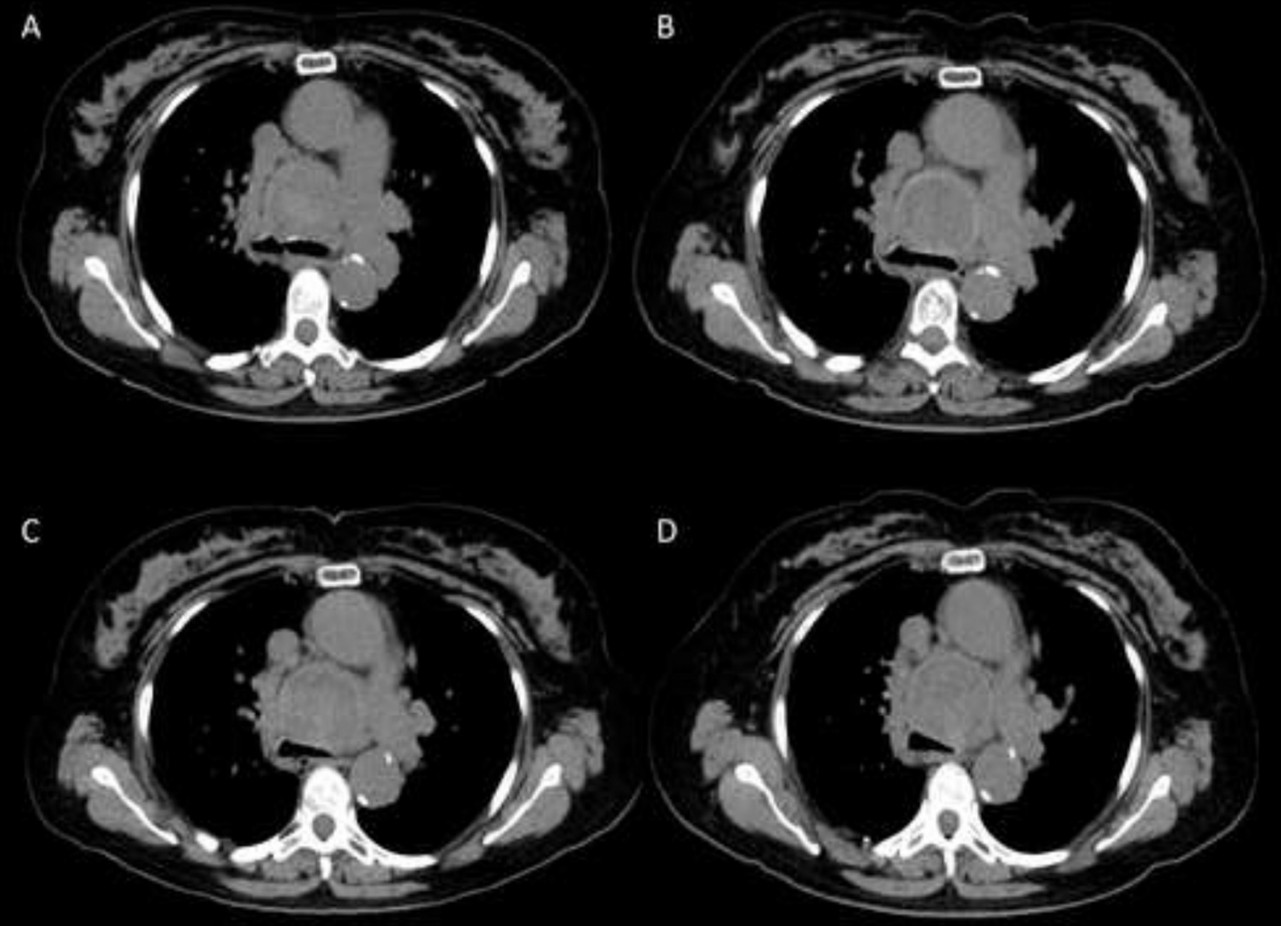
After receiving 2 y of serial imaging follow-up, patient was referred to our tertiary care center for further workup. Images from the outside institution were reviewed. (A) baseline, (B) 6-mo follow-up, (C) 12-mo follow-up, and (D) 24-mo follow-up. Examination of serial CT component of FDG PET/CT exams showed dynamic change in the internal hyperdense focus within the large round mediastinal mass throughout the examinations which was highly suggestive of a pseudoaneurysm as opposed to a malignant soft tissue mass. Further evaluation with CT angiogram was recommended.
